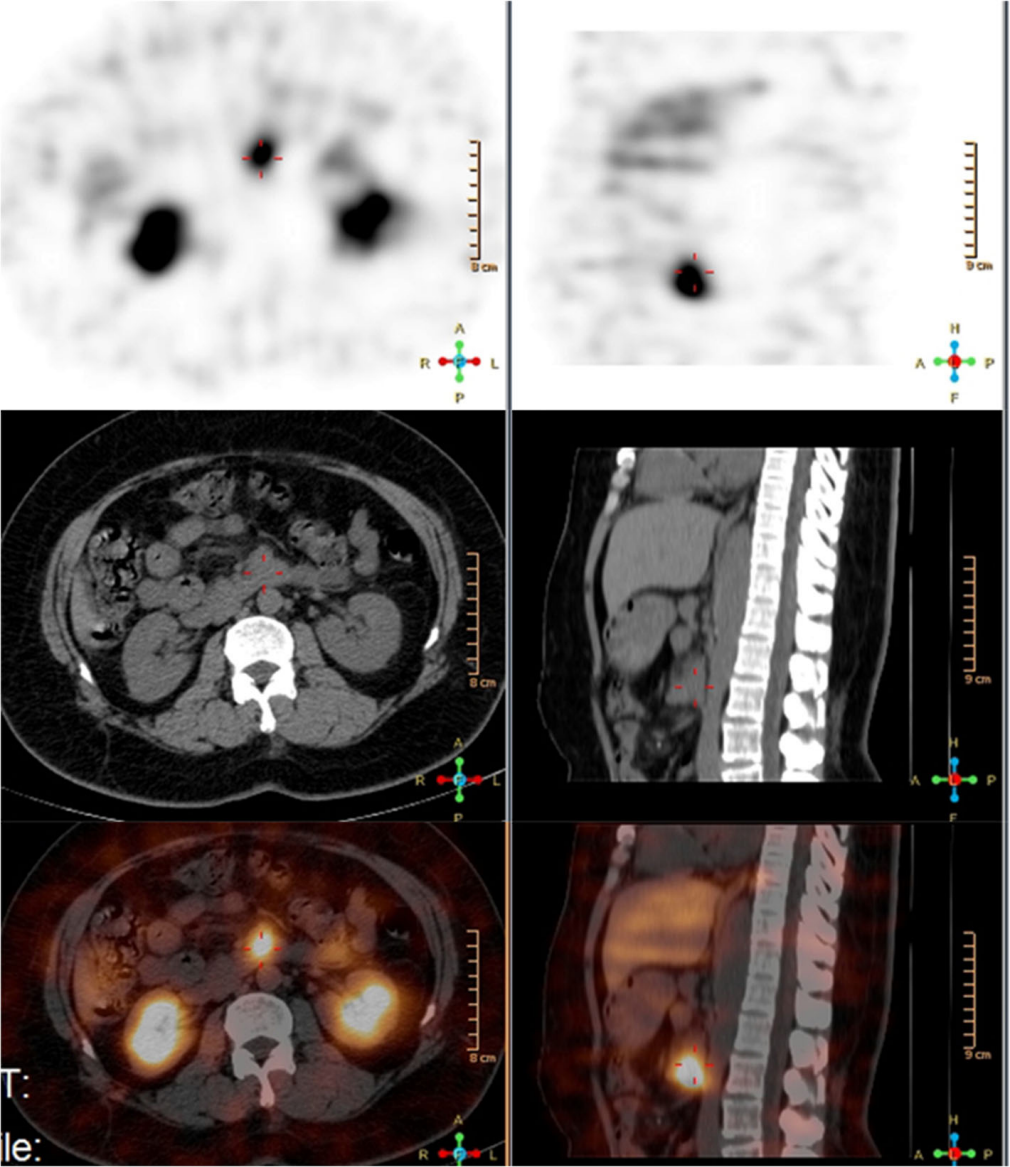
Case 1: 99mTc-HYNIC-TOC SPECT/CT shows increased radioactivity in the lesion anterior-inferior to the duodenum
